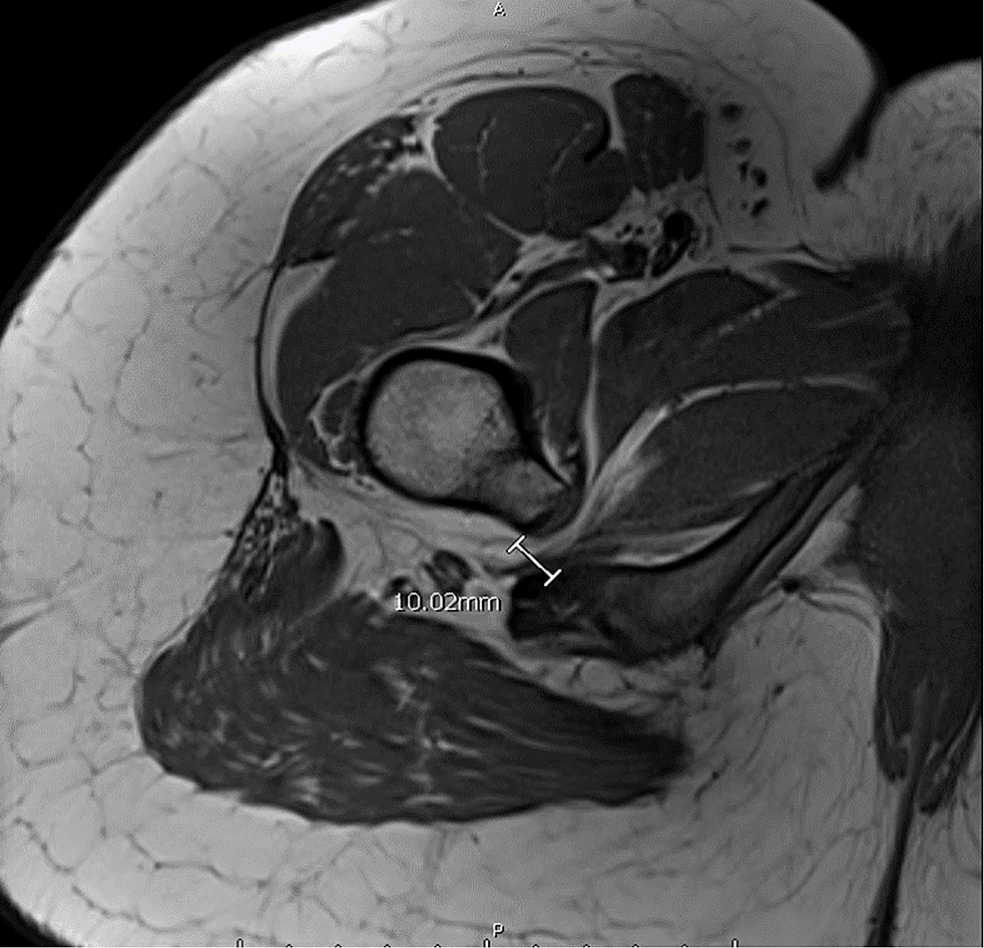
Ischiofemoral impingement is visualized on an axial T1 MRI. The distance between the lesser trochanter and the ischial tuberosity is approximately 10 mm, with evidence of fatty infiltration of the quadratus femoris muscle.
